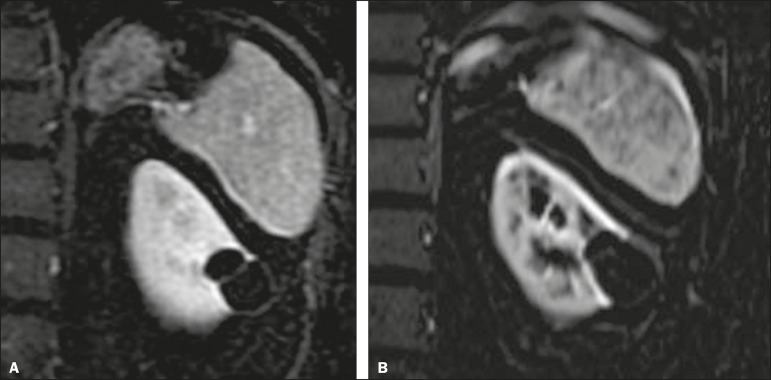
Upper abdominal MRI, in a coronal view after digital subtraction, showing
